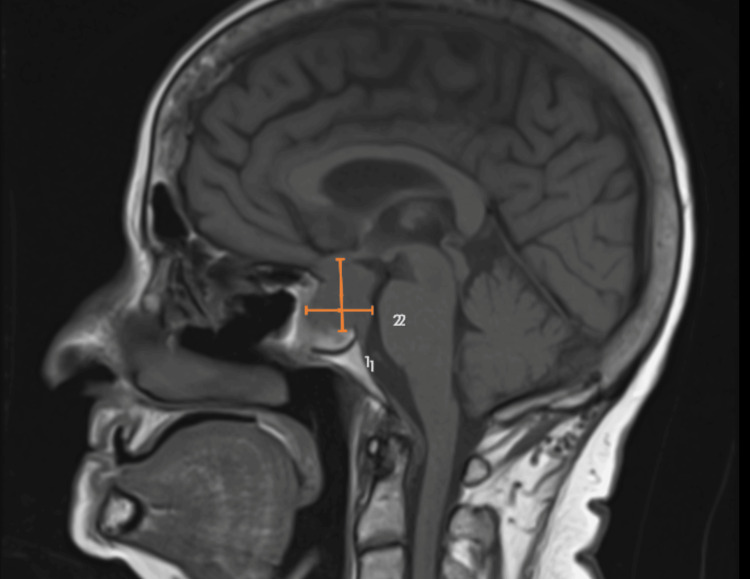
MRI of the brain (T1-weighted post-contrast sagittal image) revealing a pituitary macroadenoma with optic chiasm compression (marked by orange line 1) and probable invasion of the left cavernous sinus (marked by orange line 2). The heterogeneously enhancing sellar mass measures approximately 2.2 × 1.8 cm with suprasellar extension. MRI: magnetic resonance imaging
